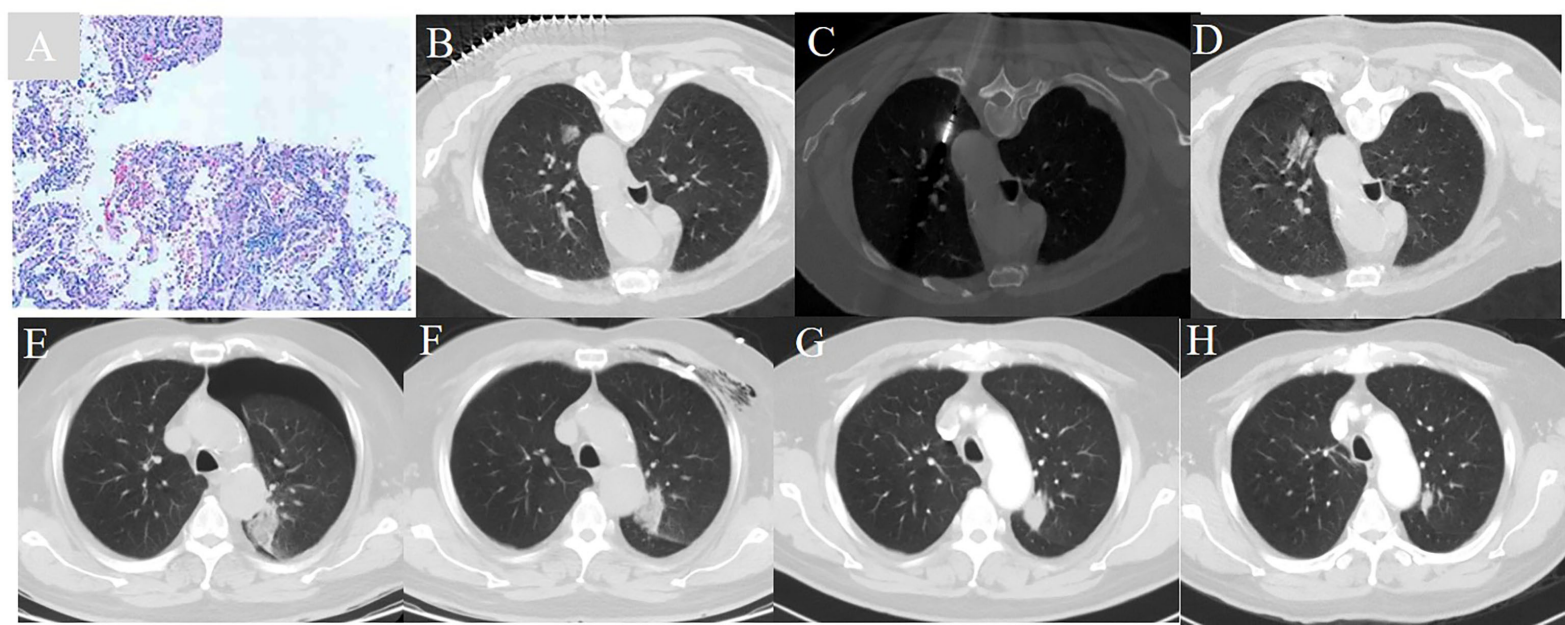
A 72 year-old man with a history of COPD and a FEV1 value of 51.2% underwent CT guided microwave ablation after pathological diagnosis of adenocarcinoma in situ. (A) Pathology verified adenocarcinoma in situ. (B) A ground glass nodule with a diameter of 1.7 cm located in the left upper lobe. (C) MWA was conducted with the power of 65 W for a total of 4.5 min. (D) The immediate CT scan showed the nodule was surpassed by the exudative change. (E) A CT scan showed moderate pneumothorax 24 hours post-ablation. (F) Pneumothorax was resolved 72 hours post-ablation. (G) The ablative zone shrank and increased in density one month post-ablation. (H) The CT ablative zone further reduced one year post-ablation.
